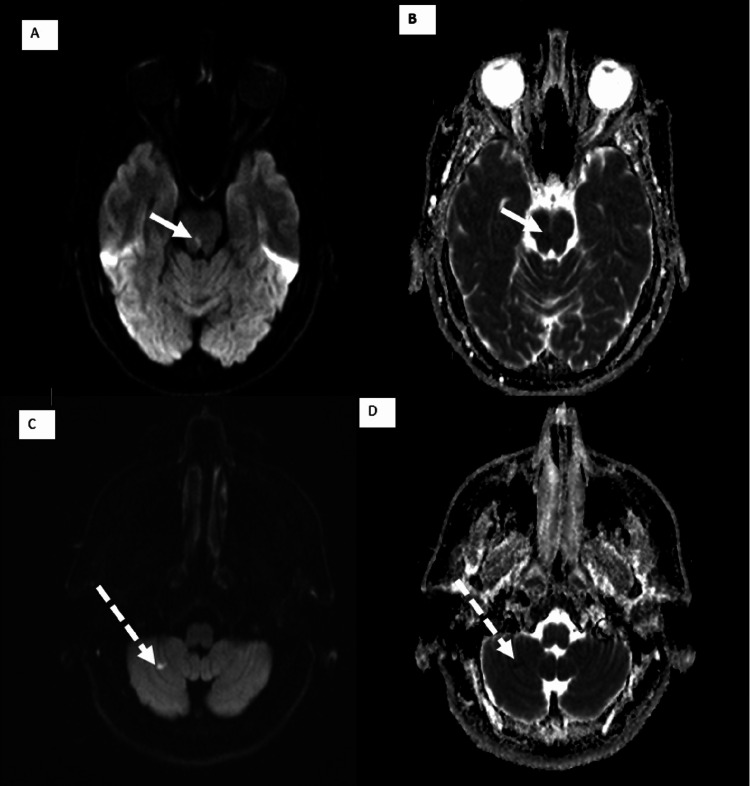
Axial MRI images (A,B) DWI and ADC showing an acute small right posterior pontine infarct (white arrows) (C,D) DWI sequence and ADC showing an acute small infarct in the right cerebellar region (dashed arrows) DWI, diffusion-weighted image; ADC, apparent diffusion coefficient
